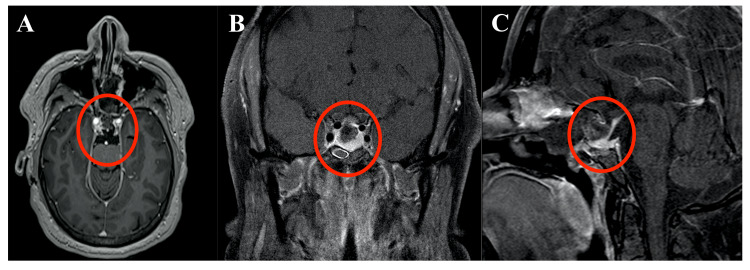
Postoperative T1-weighted MRI with gadolinium contrast demonstrates complete adenoma resection. (A) Axial, (B) coronal, and (C) sagittal plane images are displayed. MRI, magnetic resonance imaging.
